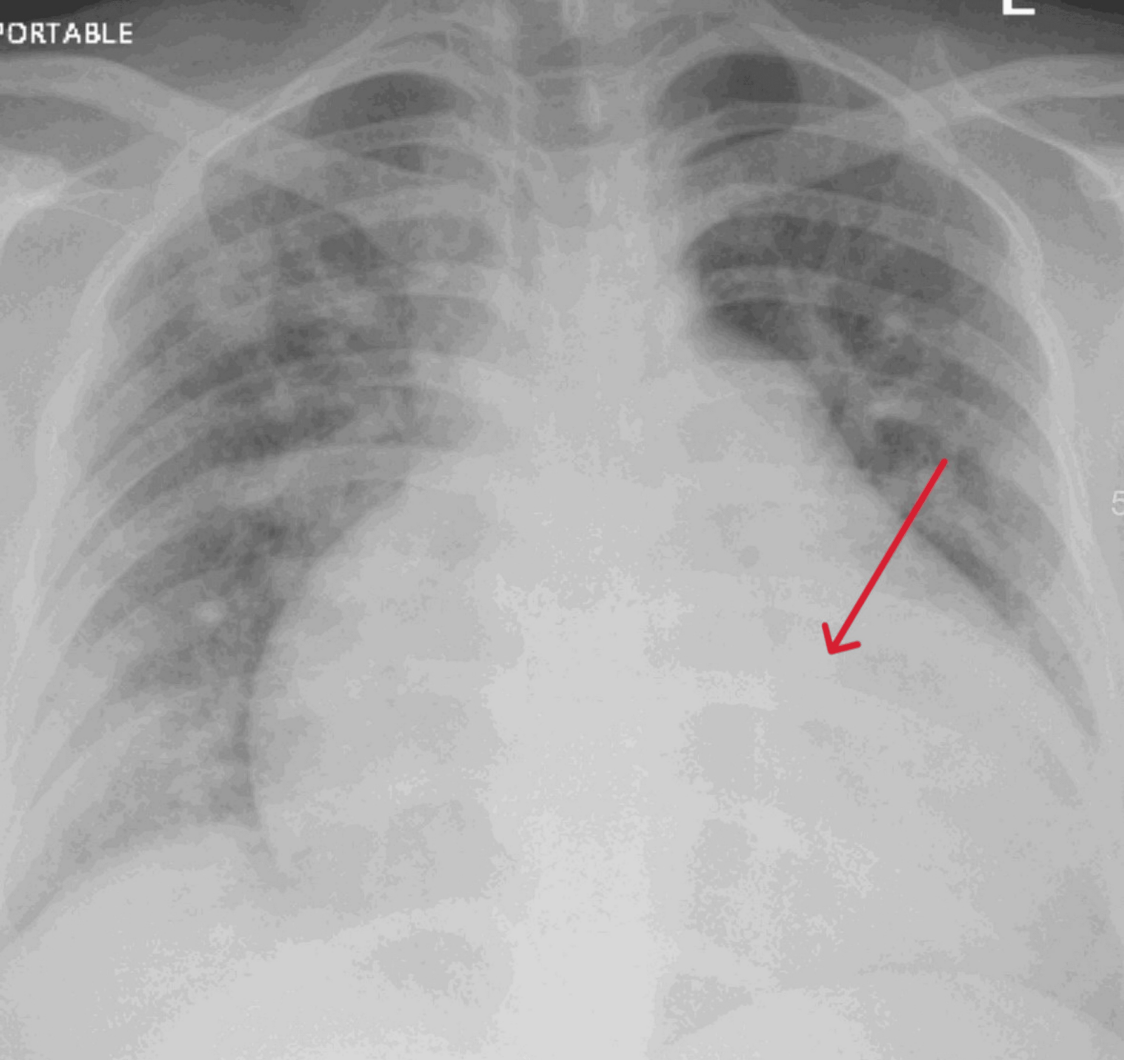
Chest X-ray, AP view, showing enlarged cardiac silhouette with pulmonary vascular congestion.
